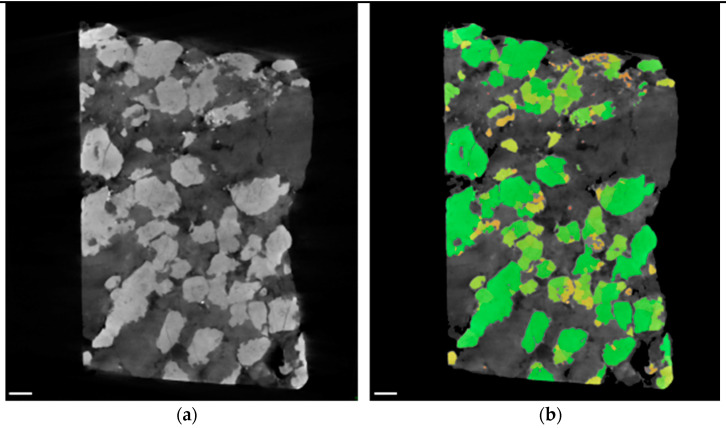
CT examination of nodular iron ore: (a) virtual cross section through reconstruction in XZ‑plane. Nodules (bright) and pores (black) are visible; (b) results of blob analysis, identified blobs are labelled green, orange and yellow (colors are related to blob size). Scale bars are 2 mm.
